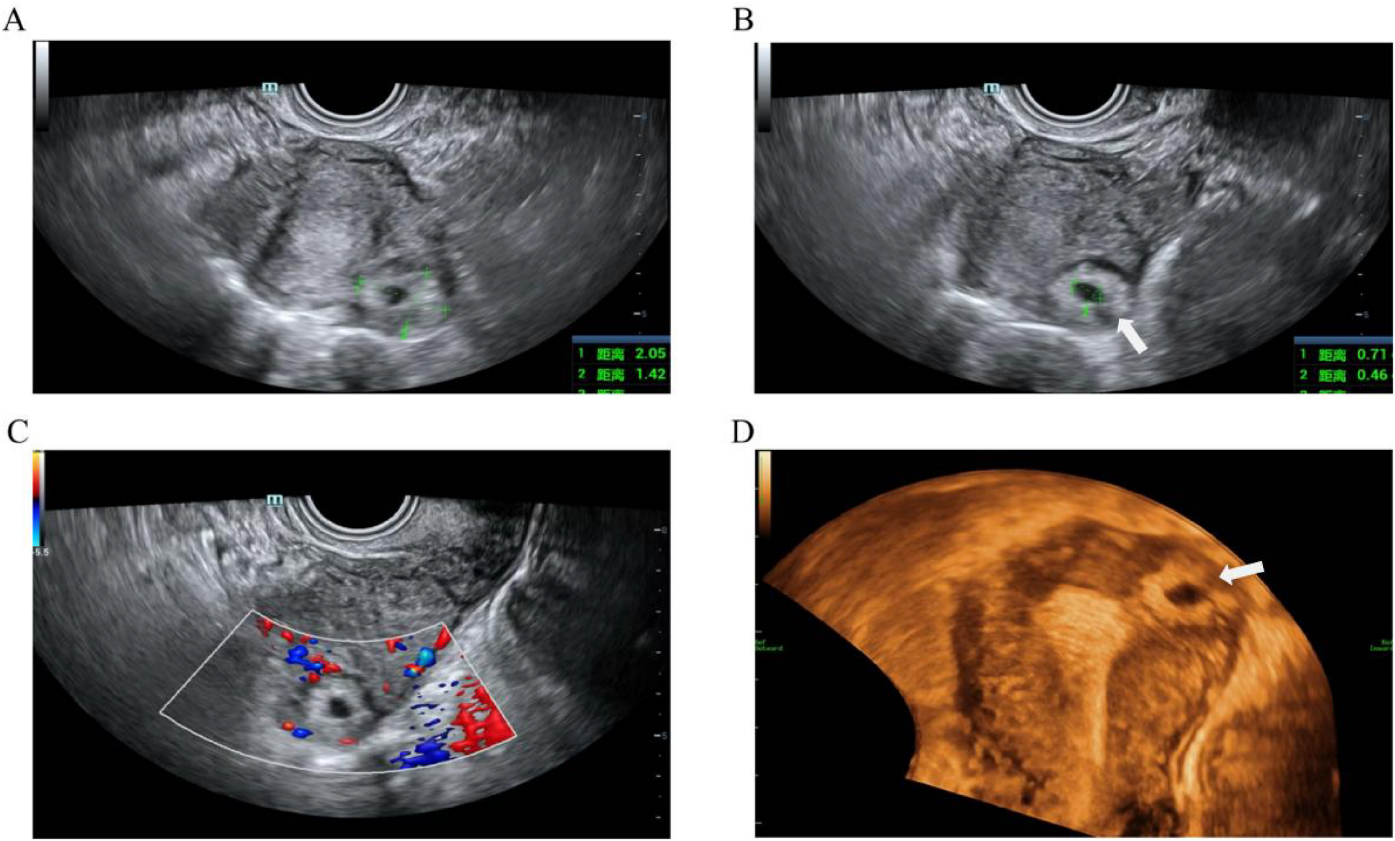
Type II angular pregnancy: (A) The two-dimensional ultrasound showed a mixed echo pattern mass measuring 21 mm × 14 mm × 18 mm in the left uterine angle, expanding toward the cornual region of the uterus. (B) A mixed echo identified a gestational sac measuring 7 mm × 5 mm × 6 mm. (C) Image of blood flow of AP in the left uterine angle. (D) The 3-dimensional ultrasound showed a mass adjacent to the endometrium, expanding toward the cornual region of the uterus. The arrow indicates a thin myometrial wall.
